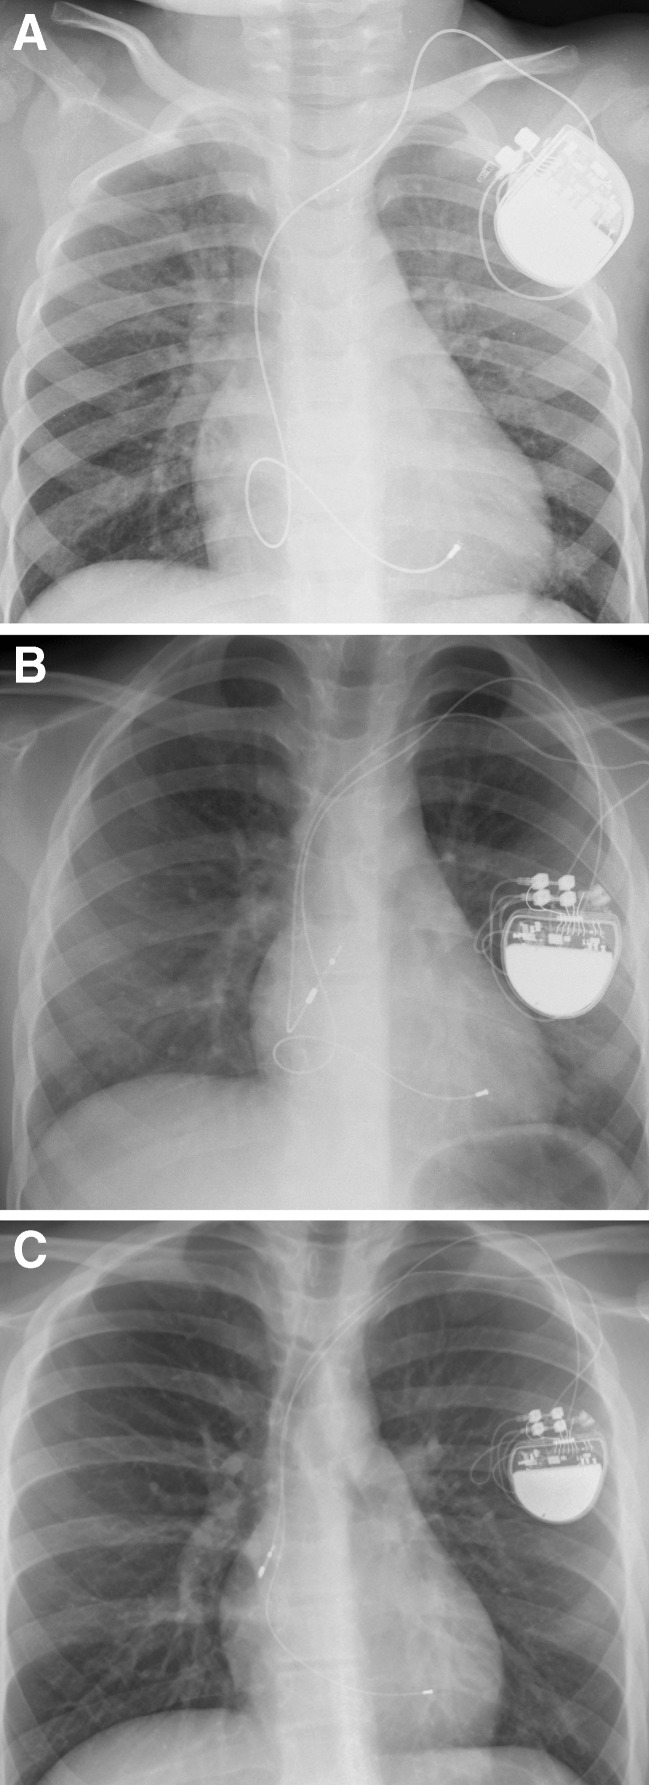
Permanent pacemaker with transvenous leads. Growth and change in a loop of an endocardial lead. A 4-year-old boy with childhood isolated nonimmune atrioventricular block underwent pacemaker implantation using a transvenous lead. Radiographs at 4 years of age (a, VVI pacing), 6 years later (b, DDD pacing), and 9 years later (c, DDD pacing). Note the change in the loop of the lead as the child grows
